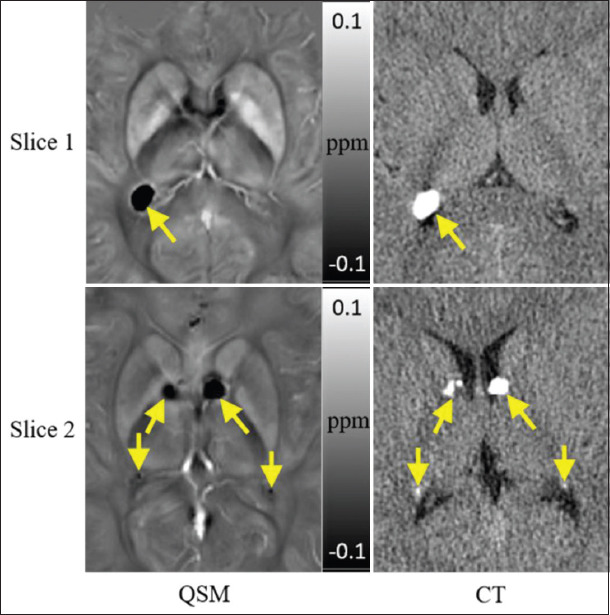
Quantitative susceptibility mapping and computed tomography showing calcified subependymal nodules from a 27-year-old male patient. Both imaging modalities could demonstrate all of the calcification.
